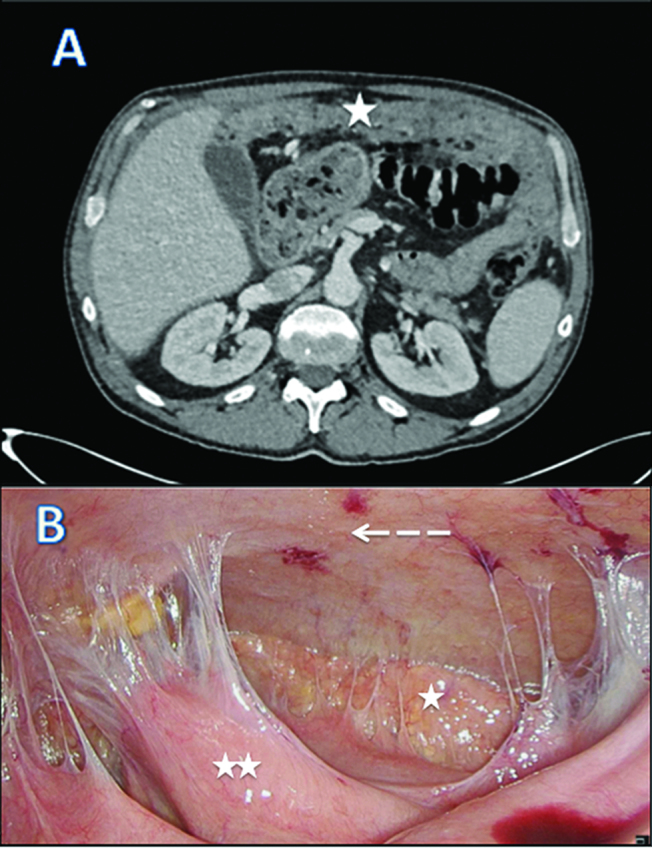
CT scan (A) and laparoscopic evaluation (B) after bi–directional chemotherapy.
